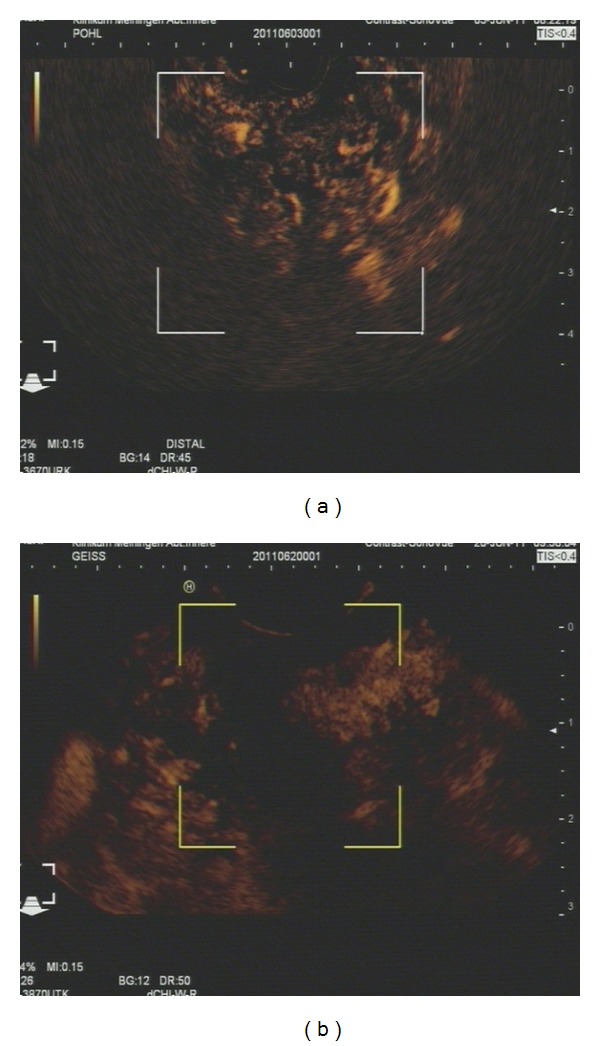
Low mechanical index contrast enhanced endosonography: (a) chronic pancreatitis with a clear enhancement of the contrast enhancer; the lesion is visible inside the markers mostly in the right upper area; all the bright visible spots are contrast enhancer signals (b) pancreatic carcinoma with a lack of contrast enhancer in the lesion; the lesion is visible within the markers; there is a black area without any contrast enhancer signals.
